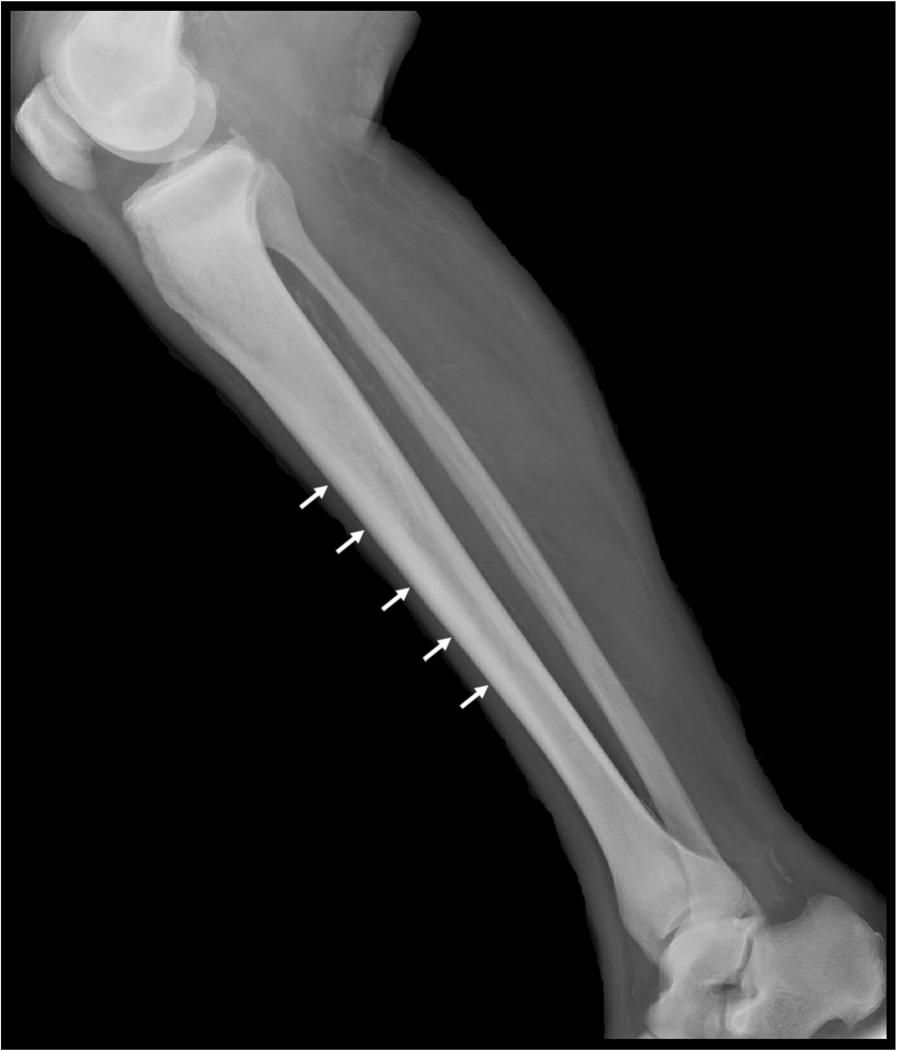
Lateral radiograph of a 62-year-old male with known primary myelofibrosis demonstrating endosteal sclerosis, the osteosclerotic pattern of myelofibrosis in the long bones (white arrows)
